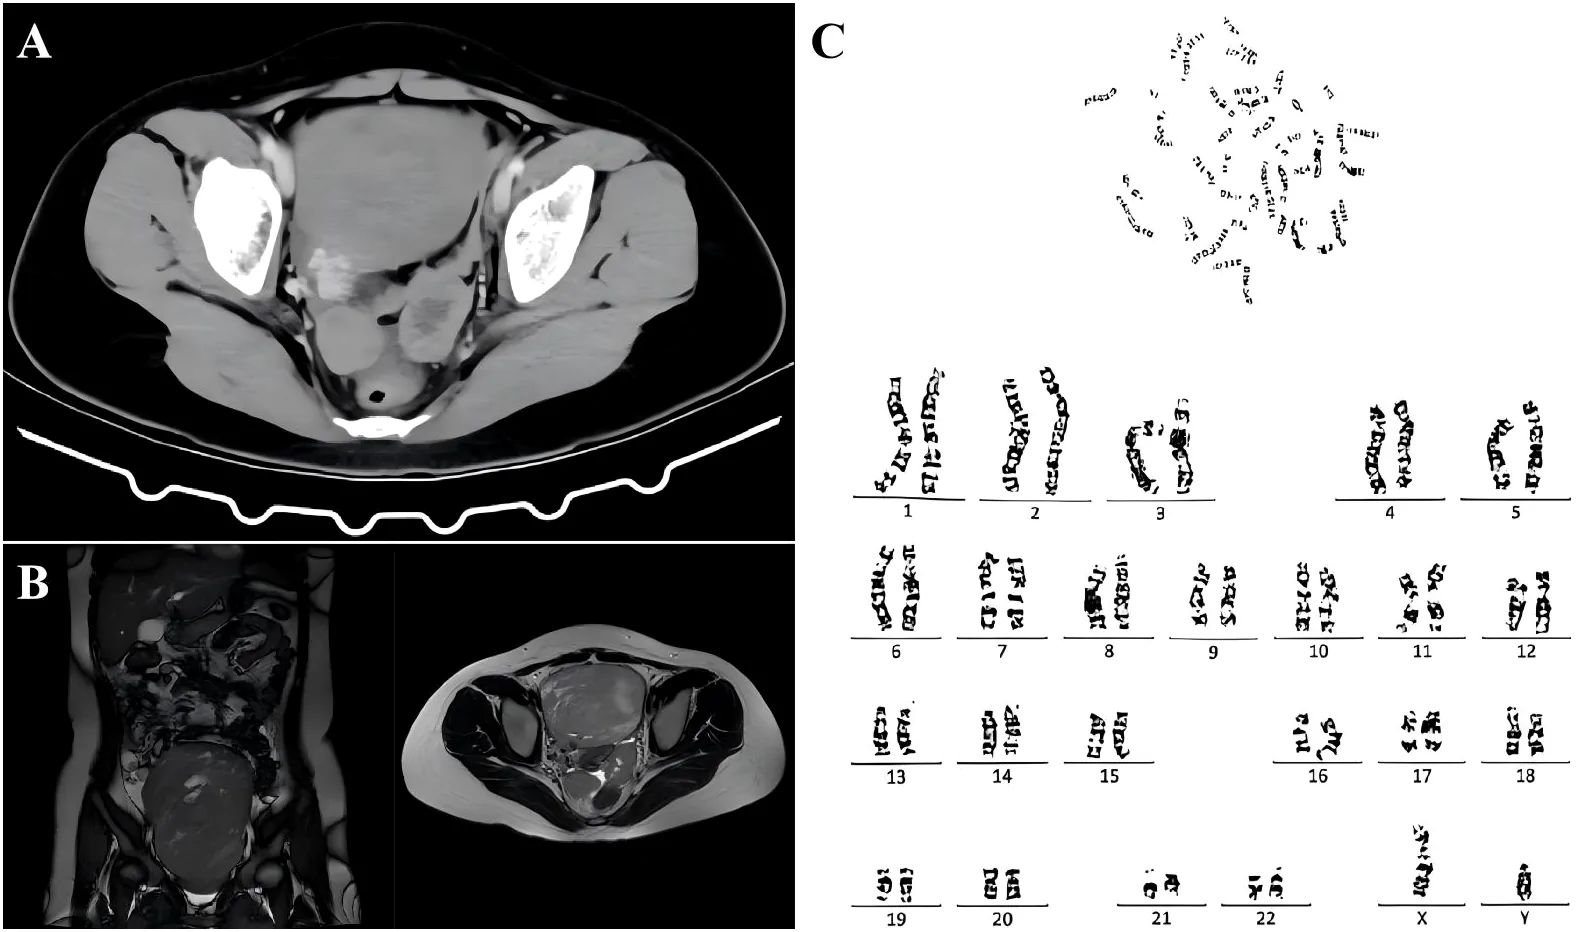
Preoperative imaging and chromosomal karyotyping results of the patient. (A) Preoperative contrast-enhanced chest and abdominal CT revealed bilateral ovarian masses highly suggestive of dysgerminoma, accompanied by a small right pleural effusion, mild intra-abdominal and pelvic effusions, and mild right hydronephrosis. (B) Coronal and axial sequences of preoperative pelvic MRI showed bilateral ovarian and abdominopelvic masses with undetermined nature, together with a small volume of pelvic fluid. (C) Chromosomal karyotyping confirmed a 46, XY karyotype.
